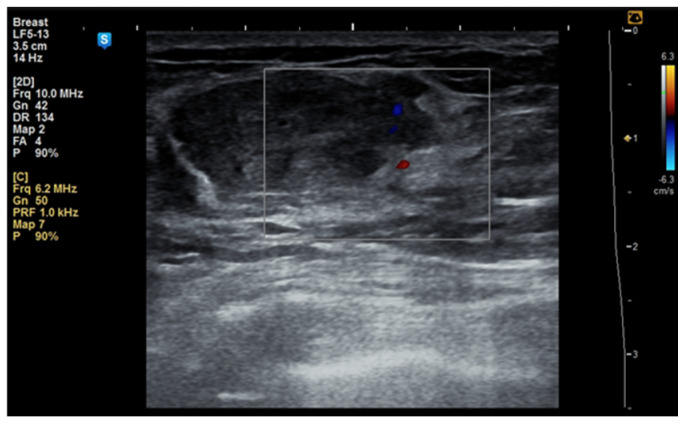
Ultrasound image of a 53-year-old woman with a right breast hamartoma with reduced vascularity, located in the lower inner quadrant.
